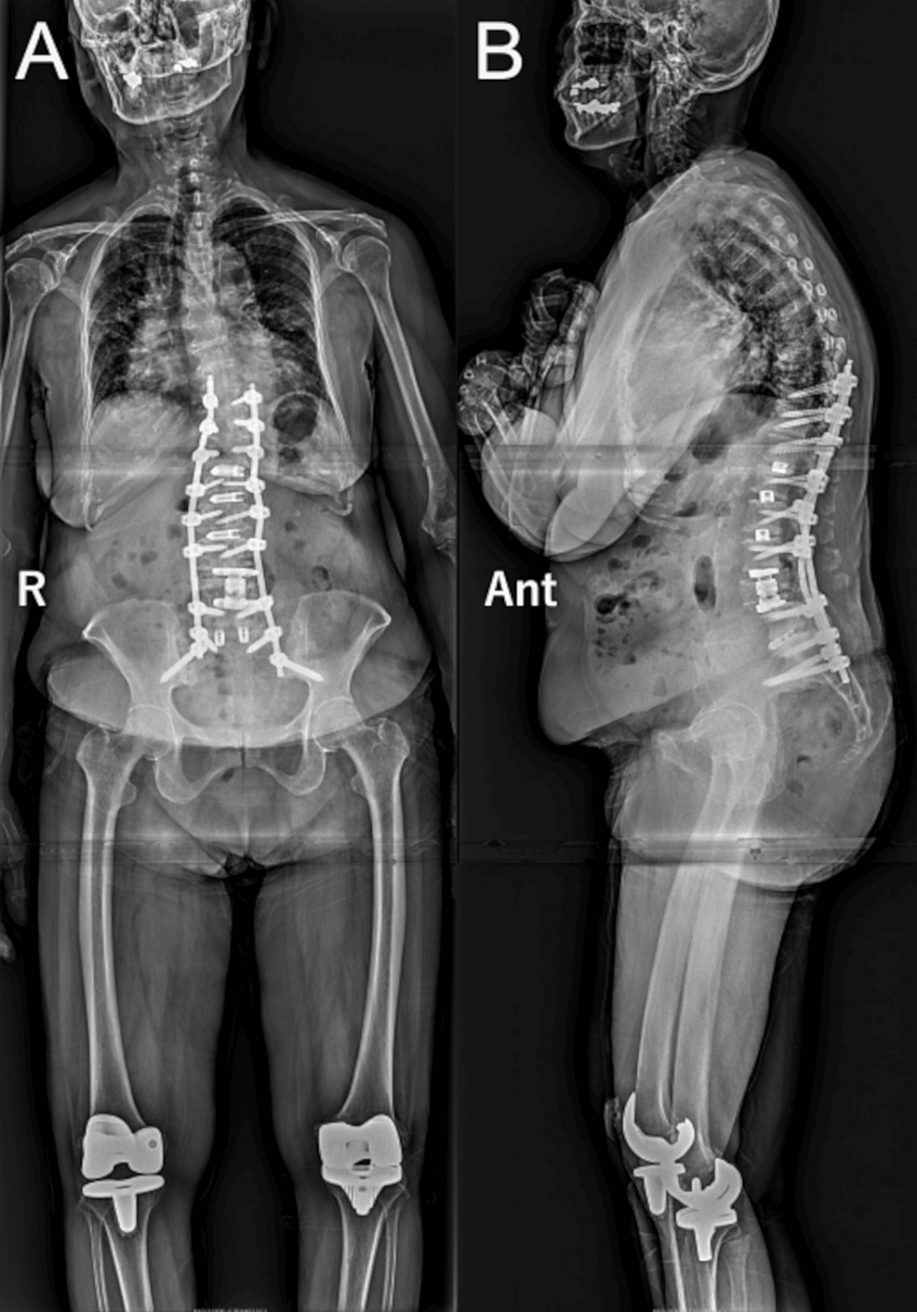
Radiographic images four months after the second operation A: anterior-posterior projection; B: lateral projection. The spinal deformity was corrected using posterior thoracic-lumbar-iliac long fusion (Ant: anterior, r: right).
